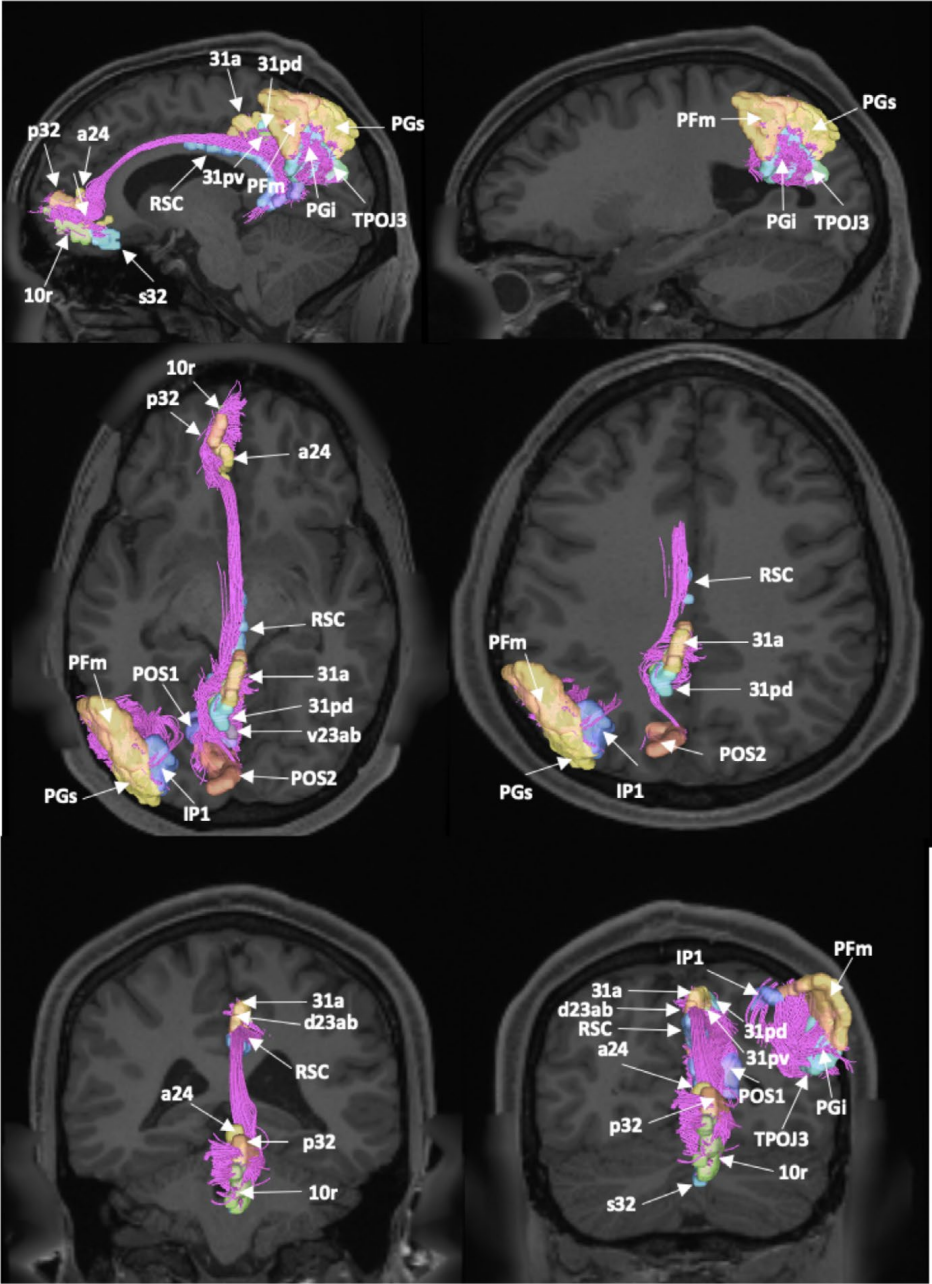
Fiber tracking analysis for the default mode network. Shown on T1‐weighted MR images in the left cerebral hemisphere. TOP ROW: sagittal sections from medial to lateral demonstrating the cingulum and its projections between the anterior and posterior cingulate clusters of the default mode network. MIDDLE ROW: axial sections from inferior to superior demonstrate the cingulum connecting the posterior cingulate and lateral parietal clusters. and the short fiber connections within the network. BOTTOM ROW: coronal sections from anterior to posterior provide another view of the cingulum and short fiber connections within the network
